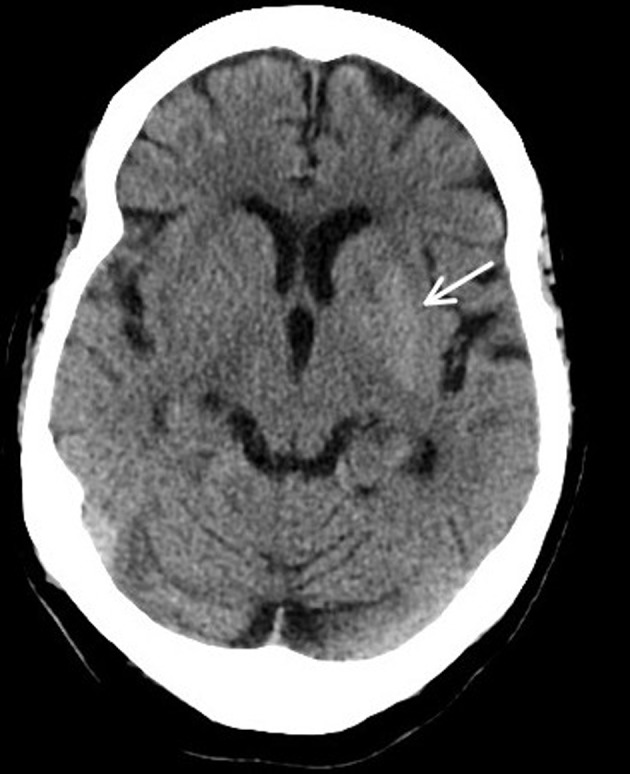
Axial CT demonstrated increased density within the caudate nuclei and left lentiform nucleus (arrow).
